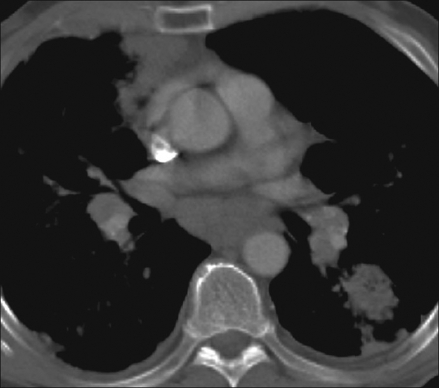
Contrast-enhanced CT (Mediastinal window settings)
